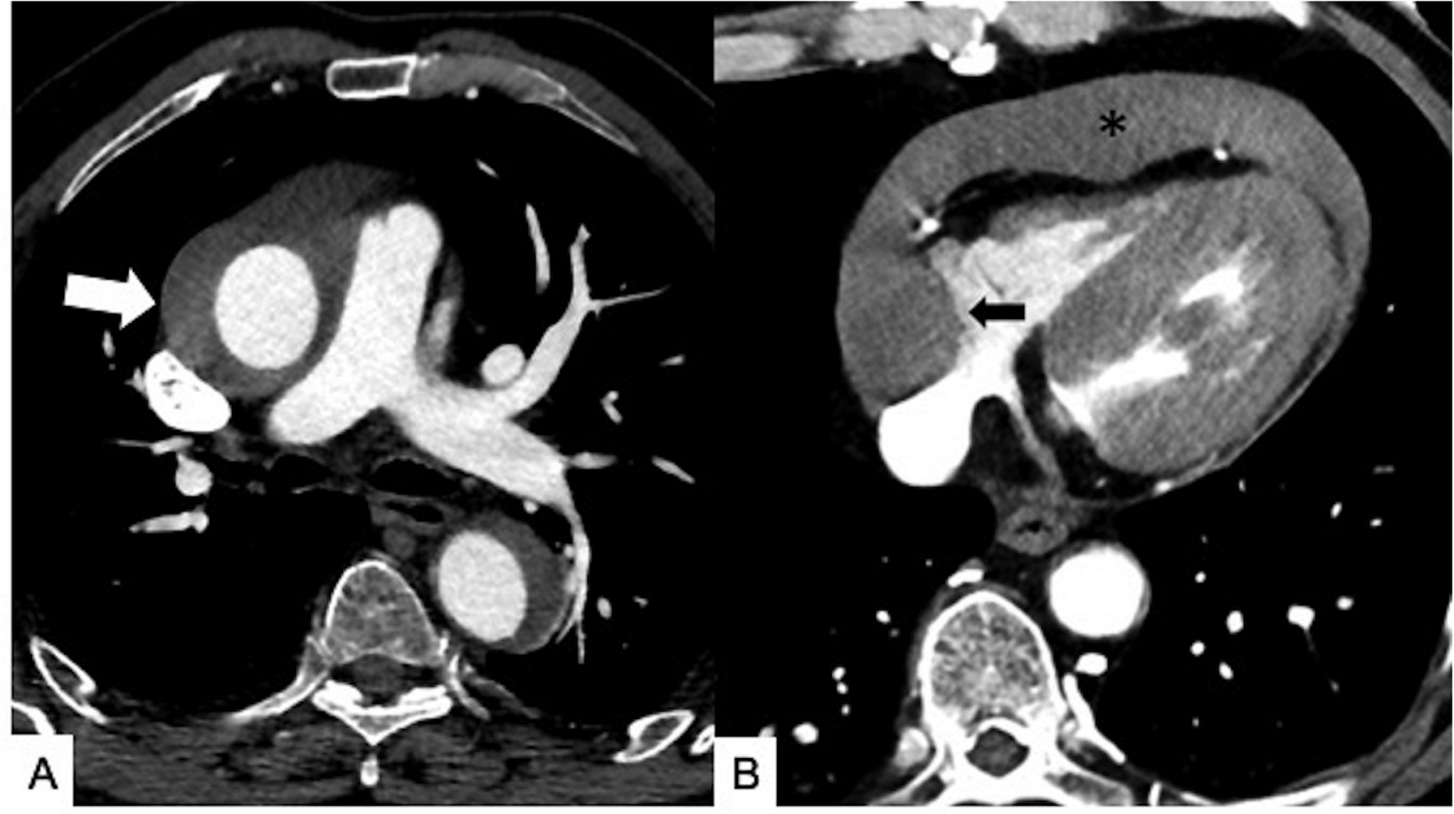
Maximal IMH thickness and pericardial effusion as poor prognostic factors in a 68-year-old male patient with acute back pain and dyspnea. (A) Axial contrast-enhanced CT scan shows a Stanford Type A IMH with hematoma thickness >10 mm (white arrow). (B) Axial contrast-enhanced CT image shows hemorrhagic pericardial effusion (asterisk) and mass effect (black arrow) on the right atrium suggesting impending cardiac tamponade.
